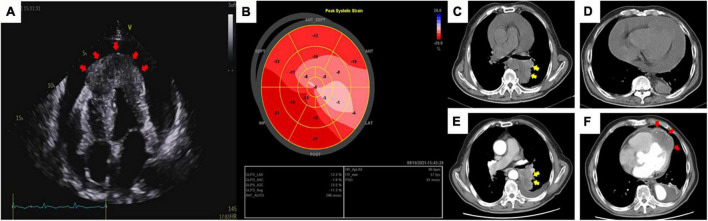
Transthoracic echocardiography (A) revealed massive pericardial effusion and a thickened and hypoechoic mass (red arrow) at the apex of the heart *via* an apical four-chamber view. Global longitudinal strain (B) showed a significantly decreased absolute value of strain in the area of the anterior and lateral walls of the heart. Routine chest CT at admission (C,D) and contrast-enhanced chest CT after pericardiocentesis (E,F) showed an irregular iso-density mass (yellow arrow) with peripheral exudation in the left lower lobe of the lung panels (C,E) and a myocardial mass (red arrow) located in the left and right ventricular apex emerging with reduced pericardial effusion panel (F).
